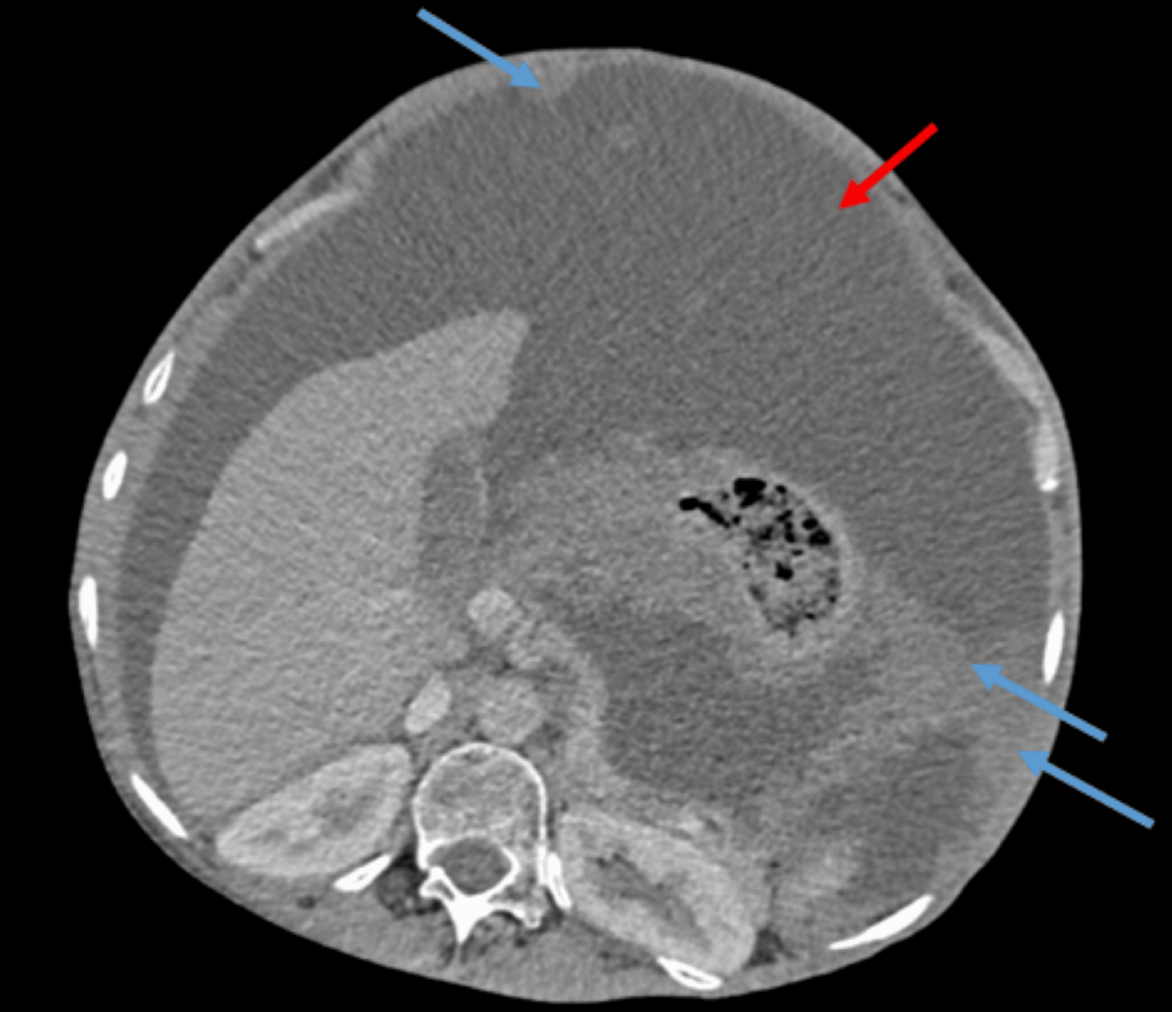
Axial view from a thoraco-abdominopelvic CT scan showing pronounced left-sided peritoneal thickening (blue arrows) and massive ascites (red arrow) This distribution supports the decision for a peritoneal-directed treatment strategy.
